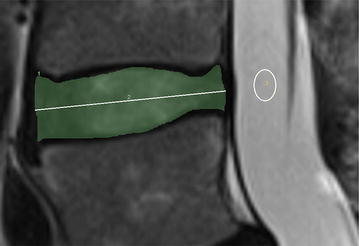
MRI tracing for quantitative measures of disc height and disc signal intensity. The *shaded region* represents the area of the disc. Disc area was defined by using the freehand region of interest measurement tool and tracing around the disc starting along the anterior longitudinal ligament, moving along the superior disc-vertebral interface, posterior longitudinal ligament and finally inferior disc-vertebral interface. Raw disc height was measured by dividing the disc area by length of horizontal line between anterior and posterior boundaries of intervertebral disc. The *elliptical region* represents the cerebrospinal fluid reference sample
